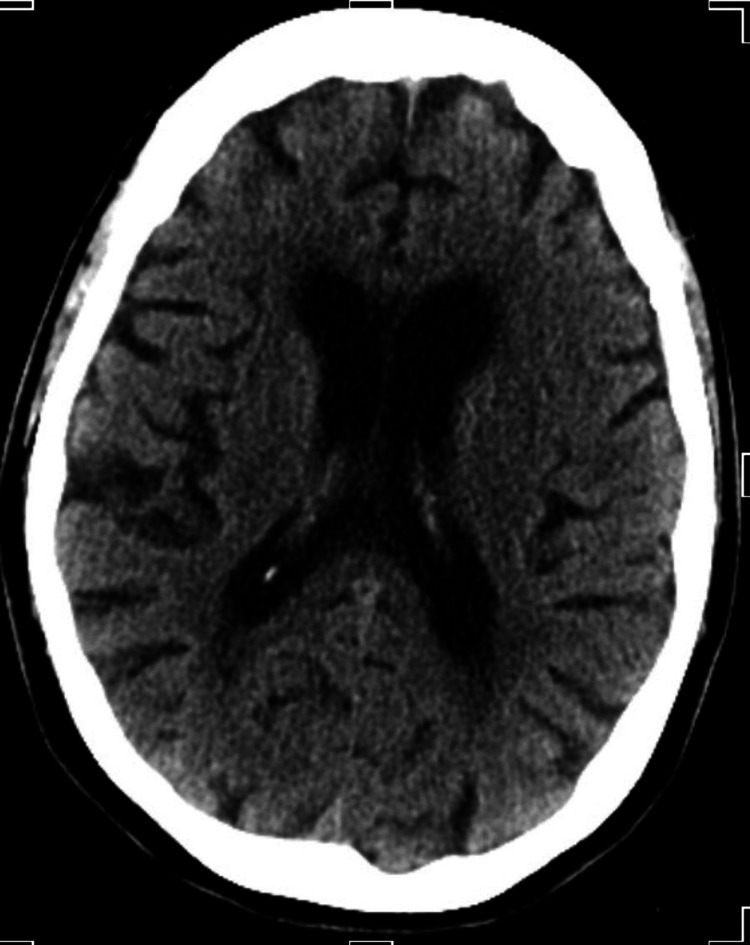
CT head without contrast showing no stroke.
